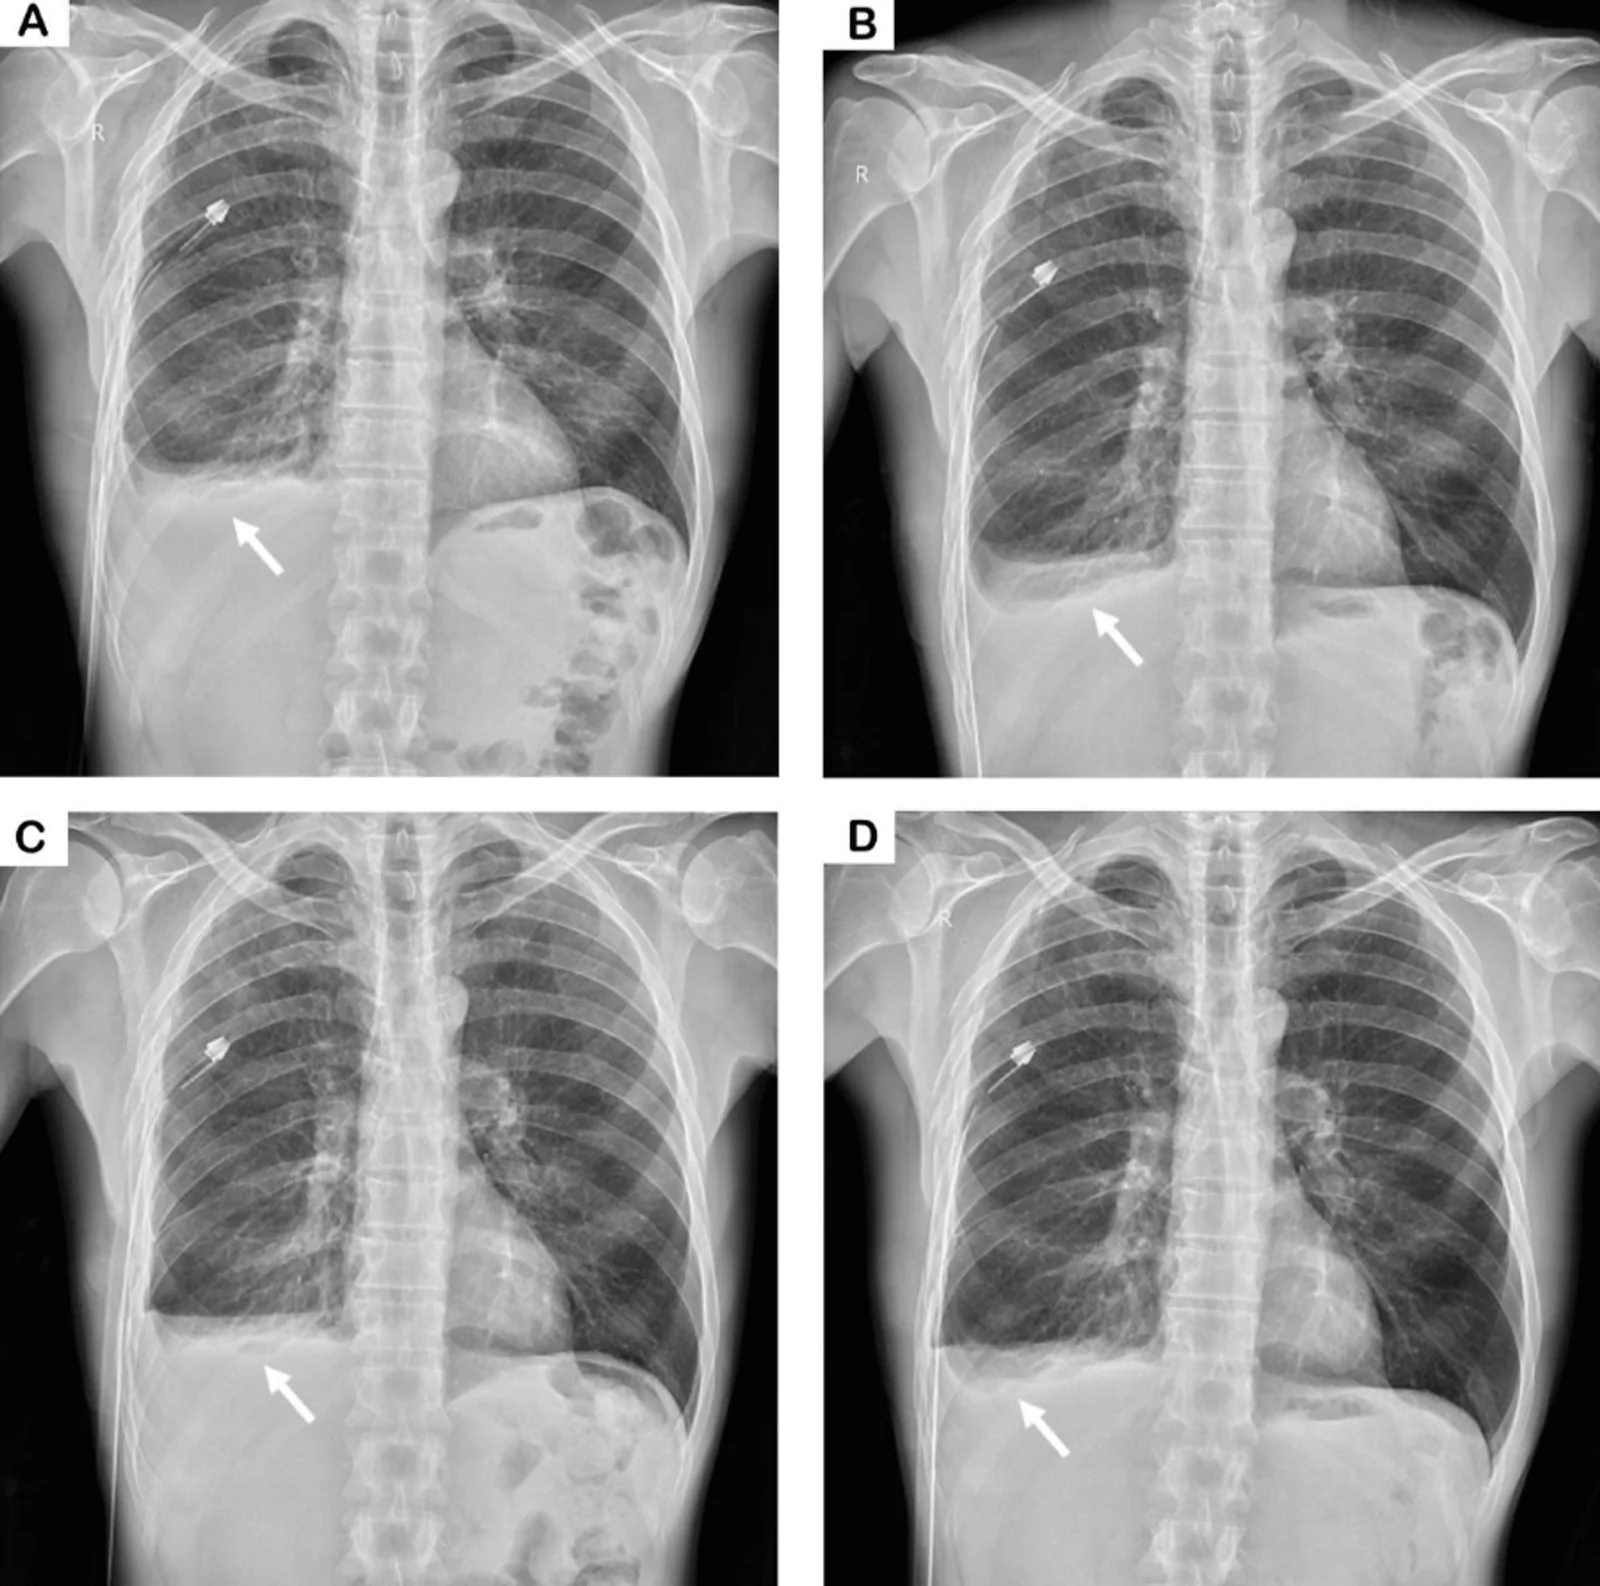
Serial daily follow-up anteroposterior chest radiographs. (A) Day three of admission: Post-drainage radiograph reveals the chest tube in situ with residual basilar fluid (white arrow). (B) Day four of admission: Notable decrease in pleural fluid volume (white arrow) with corresponding expansion of the right lung. (C) Day five of admission: Minor re-accumulation of dependent pleural fluid (white arrow) with a stable air-fluid interface. (D) Day six of admission: Final daily radiograph prior to chest tube removal demonstrates minimal residual pleural thickening at the costophrenic angle (white arrow) with near-complete resolution of the hemopneumothorax.
